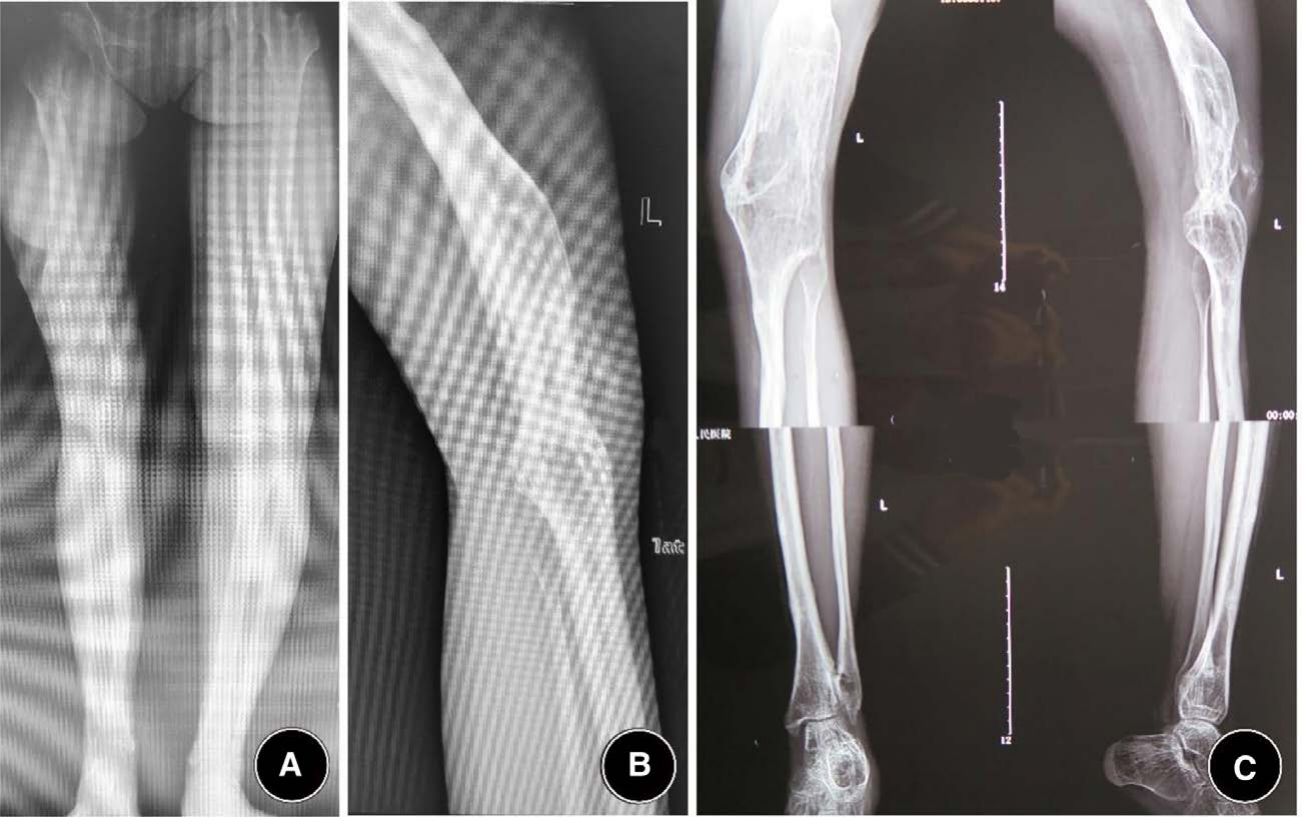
X-ray at 7.5 years postsurgery showing significant improvements in bone density and femoral/tibial diameter. (A) Standing anteroposterior radiograph showing increased trabecular density and cortical thickening of the femur and tibia. (B) Standing lateral radiograph confirming remodeling with increased cross-sectional diameters of the femur and tibia. (C) Magnified anteroposterior view.
